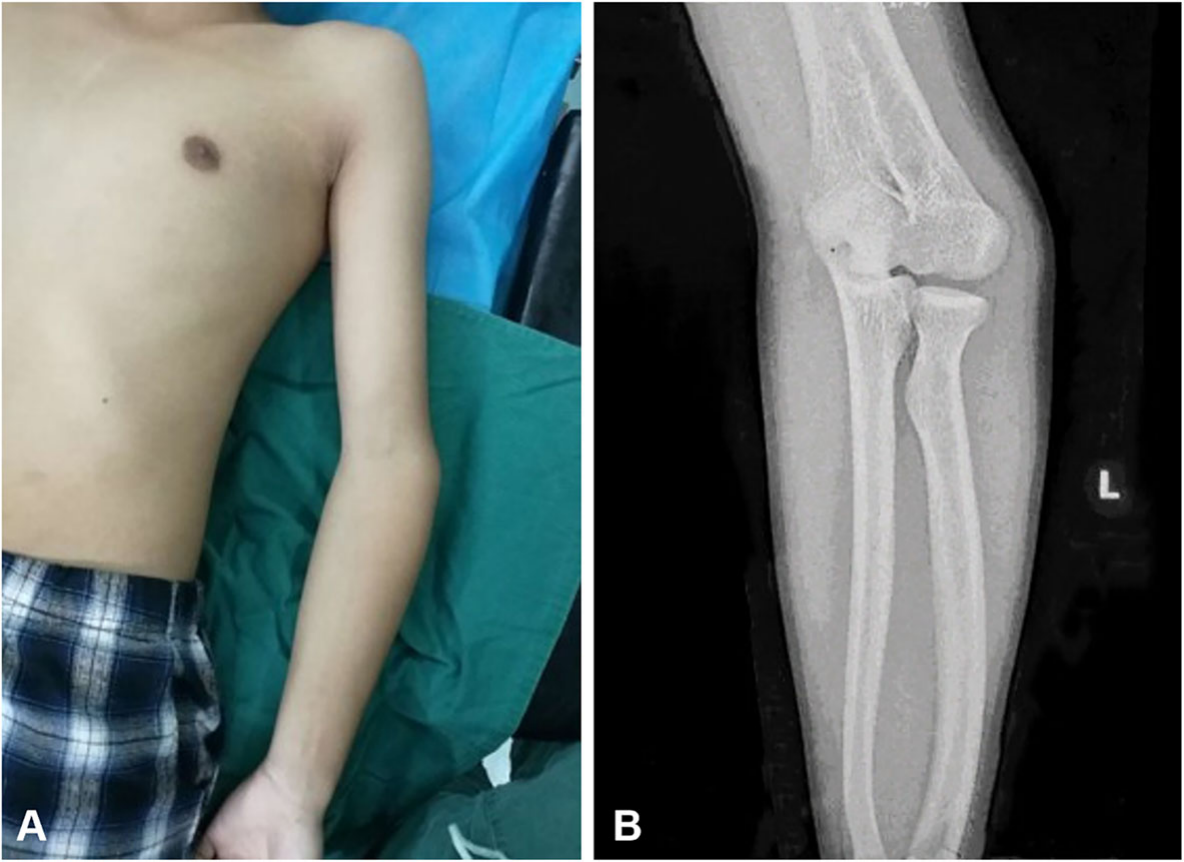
Preoperative appearance and radiographs of a 13-year-old boy with cubitus varus deformity. a Preoperative appearance. b Preoperative X-rays of the deformed side
